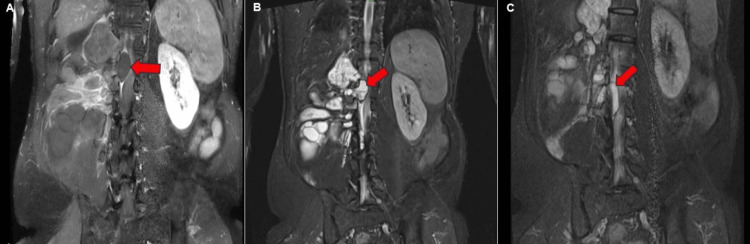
Magnetic resonance imaging of T1-weighted images with post-contrast enhancement and short tau inversion recovery images in the coronal section. (A) Post-contrast T1-weighted magnetic resonance imaging shows thin peripheral enhancement of the cysts (red block arrow). (A-C) Intraspinal extension causing the left lateral displacement and compression of the spinal cord (red block arrow). (B, C) Short tau inversion recovery magnetic resonance images showing cysts extending through the neural foramina, with intraspinal extension causing cord compression (red block arrow).
